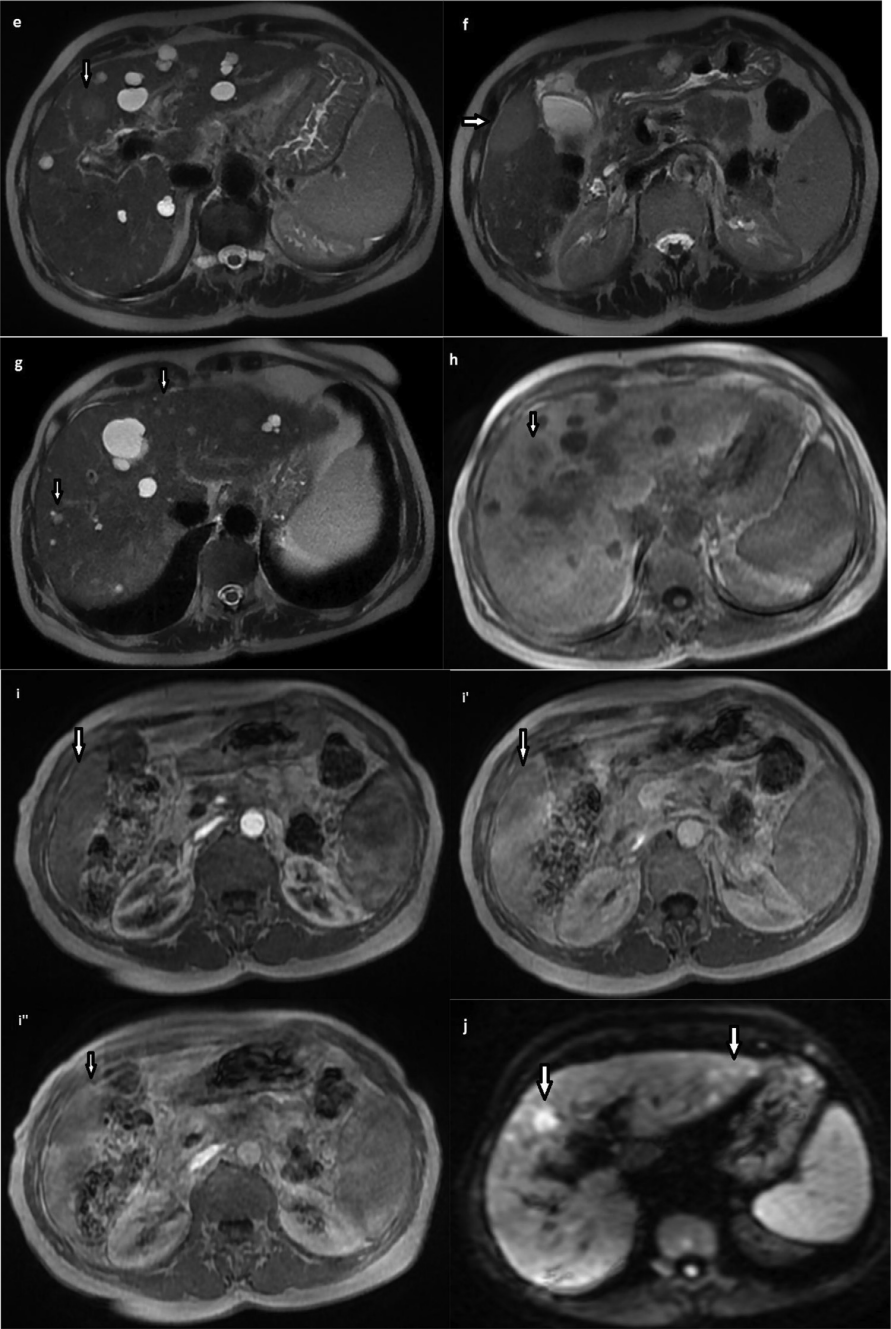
liver MRI on T2-WS (E, F, G), precontrast T1 WS (H), post contrast T1 WS (i:arterial phase, i’:portal phase, i’’:late phase), (I) and diffusion (DWI b = 800 sec/mm2) (J) showing nodular lesions nodular lesions isointense on T1 weighted sequences (WS), with intermediate signal on T2 WS, with late gadolinium enhancement on the periphery, hyperintensity on DWI and hypointensity on apparent diffusion coefficient map, suggesting restricted diffusion.
